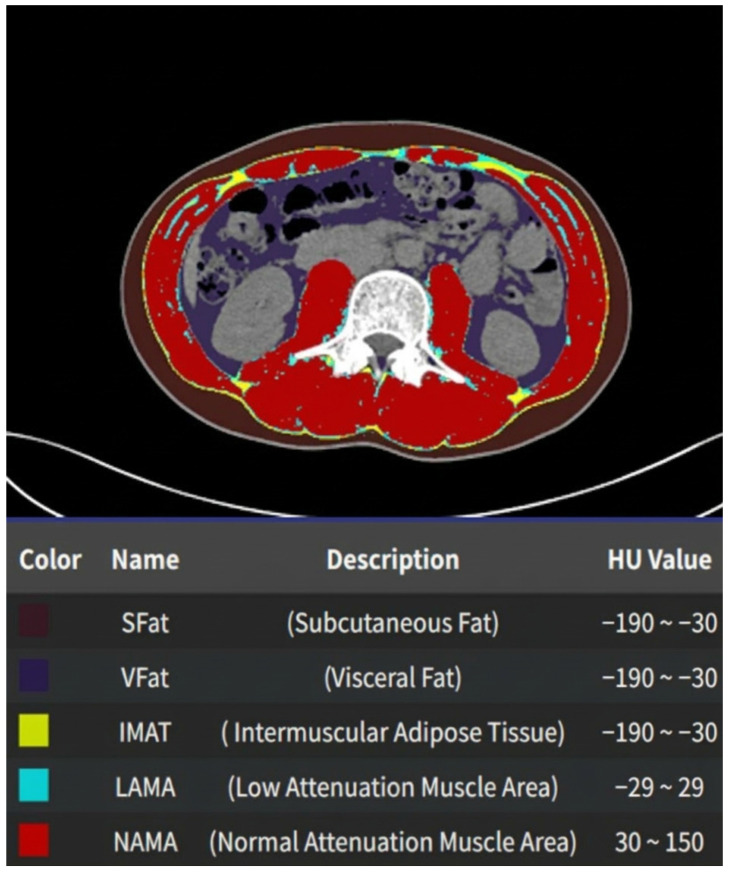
AI-based automated body composition analysis at the L3 vertebral level on computed tomography. Representative axial abdominal computed tomography (CT) image at the level of the third lumbar vertebra (L3) showing automated AI-based segmentation of body composition components. Skeletal muscle and adipose tissue compartments were identified and color-coded according to predefined Hounsfield unit (HU) thresholds. Subcutaneous fat (SFat), visceral fat (VFat), and intermuscular adipose tissue (IMAT) were defined within the range of −190 to −30 HU. Skeletal muscle was subdivided into low attenuation muscle area (LAMA; −29 to 29 HU), representing lipid-infiltrated muscle, and normal attenuation muscle area (NAMA; 30 to 150 HU), representing high-quality contractile muscle. Skeletal muscle area (SMA) was calculated as the sum of LAMA and NAMA, and skeletal muscle density (SMD) was derived as the mean HU value of the total segmented muscle area.
